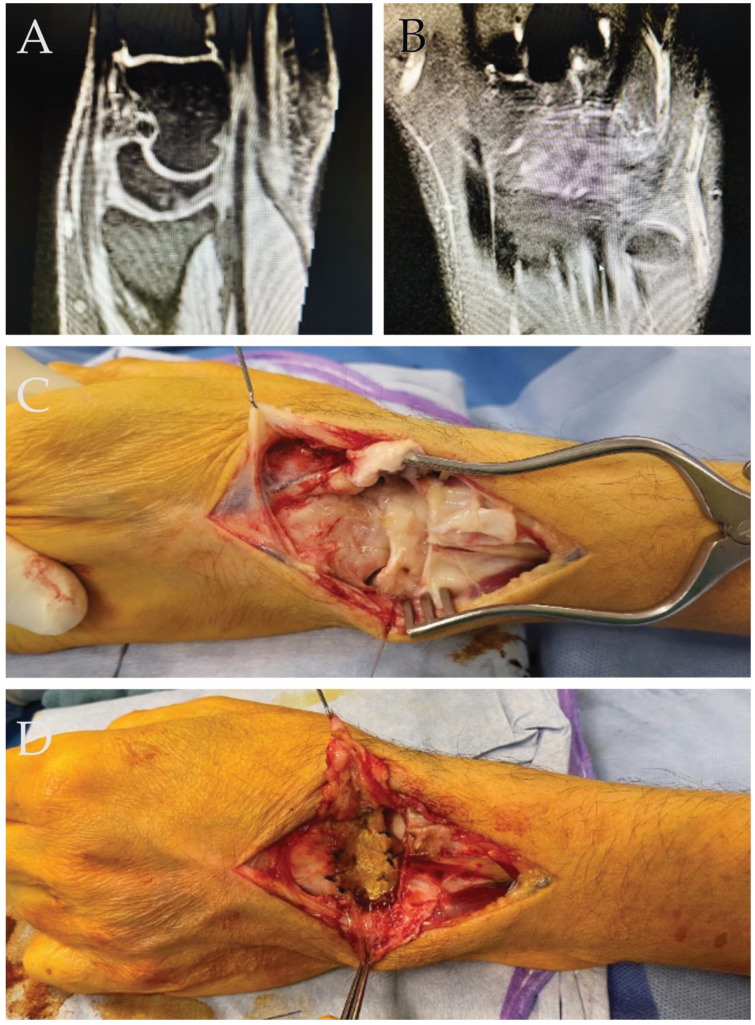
(A) Lateral view of the wrist, indicating clear “palmar intercalated segmental instability” (PISI) observed in the MRI. (B) AP view of the mucoid-degenerated dorsal intercarpal ligaments. (C) Intraoperative perspective of the wrist revealing intercarpal instability. (D) Intraoperative image post-reconstruction of the dorsal intercarpal ligaments using a collagen hADM membrane.
